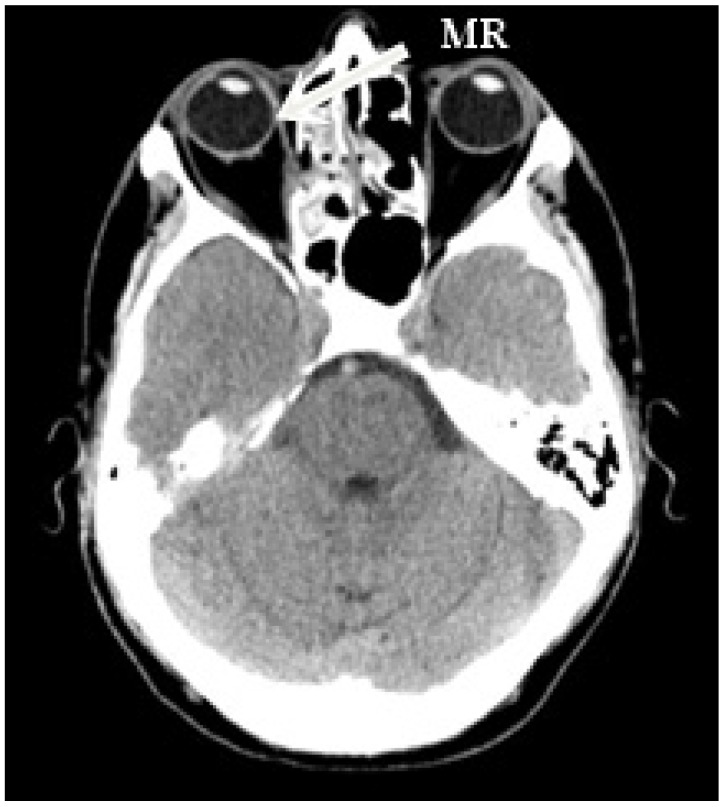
Patient 7. Axial computed tomography scan demonstrating defect in right medial orbital wall. The medial rectus was displaced medially and found adherent to the affected site, partially entrapped with defect in the lamina papyracea.
